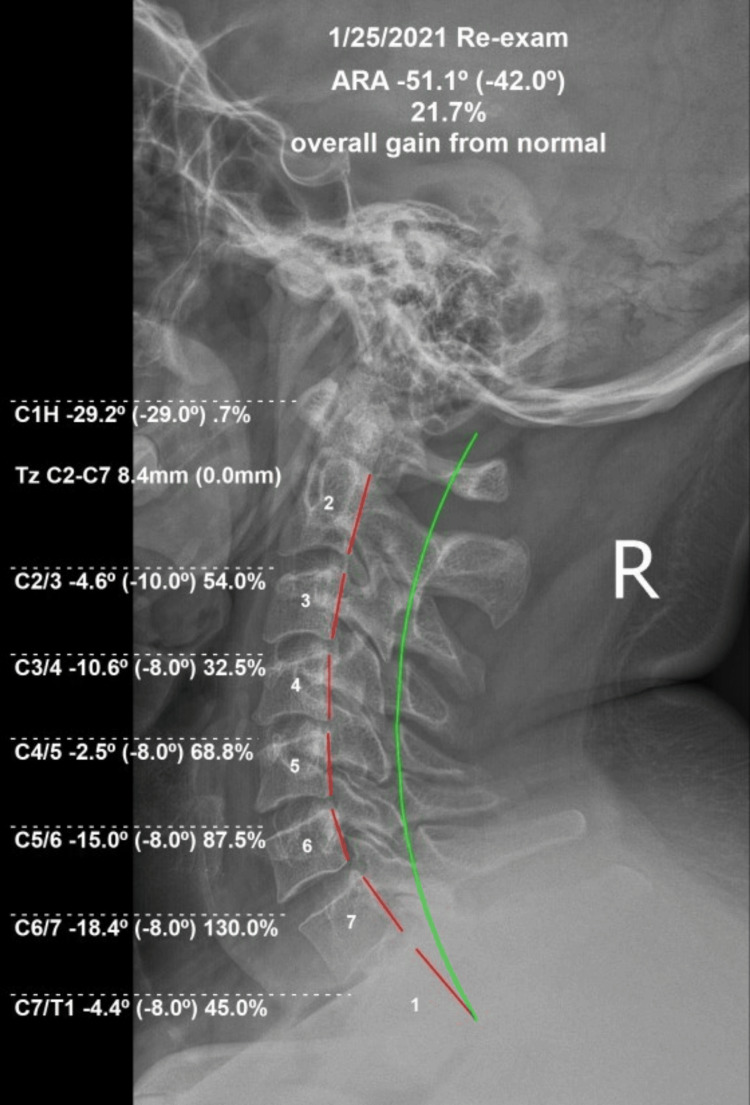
Post Treatment Lateral Cervical Radiograph The dashed red line demonstrates the restored cervical lordosis following treatment measured with the Harrison Posterior Tangent Method.  The green line represents ideal cervical lordosis.
